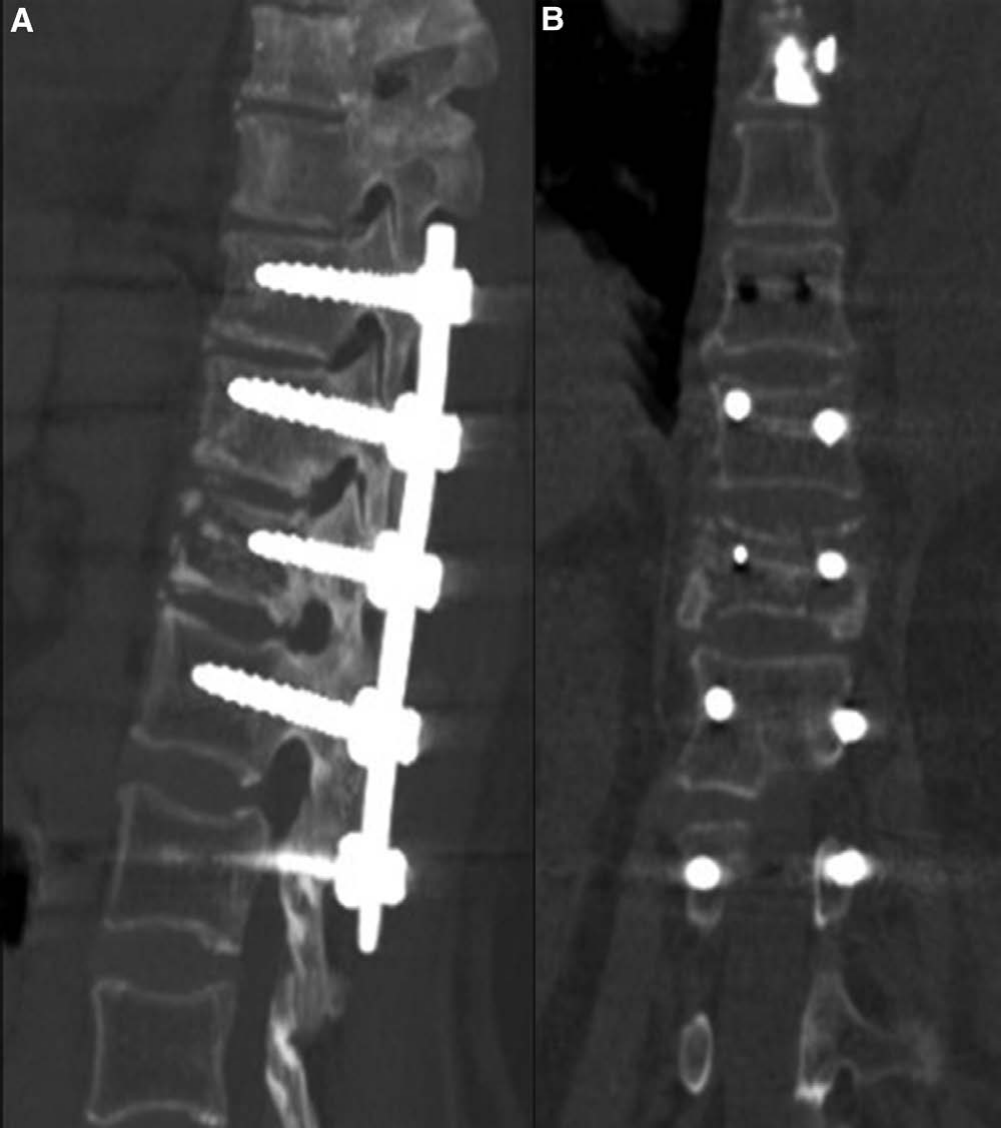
The images for T12 vertebral body treated by injured vertebral body screw fixation combined with intertransverse process grafting. A, B: Axial (A) and coronal (B) CT scans in T12 vertebral body. CT = computer tomography.
